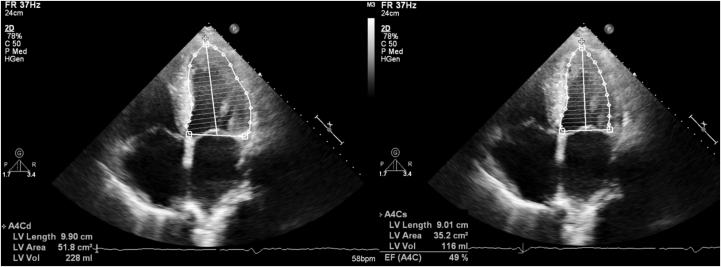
Echocardiogram (Diastolic Image on the Left, Systolic Image on the Right) Demonstrating a Left Ventricular Ejection Fraction of Approximately 45%
